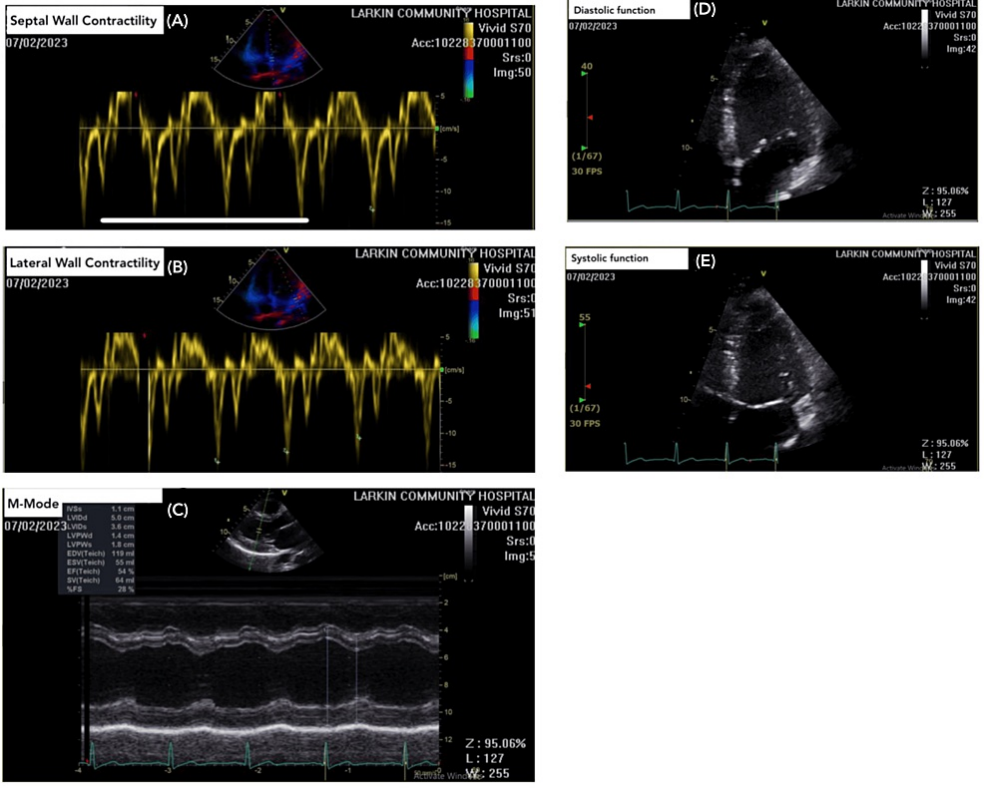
Transthoracic echocardiogram results of a 25-year-old male patient presenting with elevated troponins and complaints of chest pain (A) Septal wall view revealed mild asymmetric left ventricular hypertrophy; (B) Lateral wall view revealed left ventricular filling pattern is normal for age, normal left ventricular systolic function, and normal LVEF of 55%. (C) Motion-mode view shows aortic valve is grossly normal in structure. (D) Diastolic view shows mitral valve and tricuspid valve are grossly normal in structure. Mild mitral regurgitation. (E) Systolic view revealed mild tricuspid regurgitation with an RVSP of 25 mmHg and trace-to-mild pericardial effusion. The aortic root is normal in size. LVEF, left ventricular ejection fraction; RVSP, right ventricular systolic pressure
